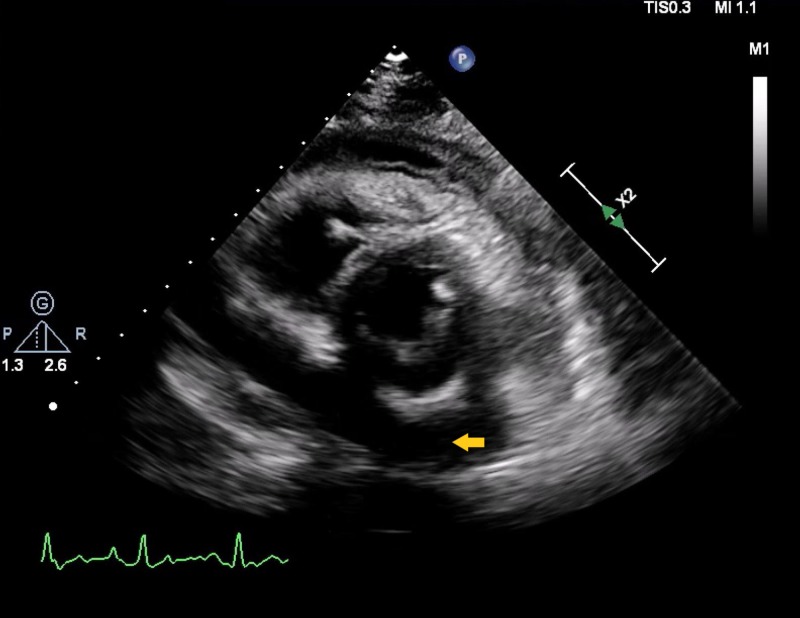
Arrow showing circumferential pericardial effusion in parasternal short axis view of transthoracic echocardiogram
